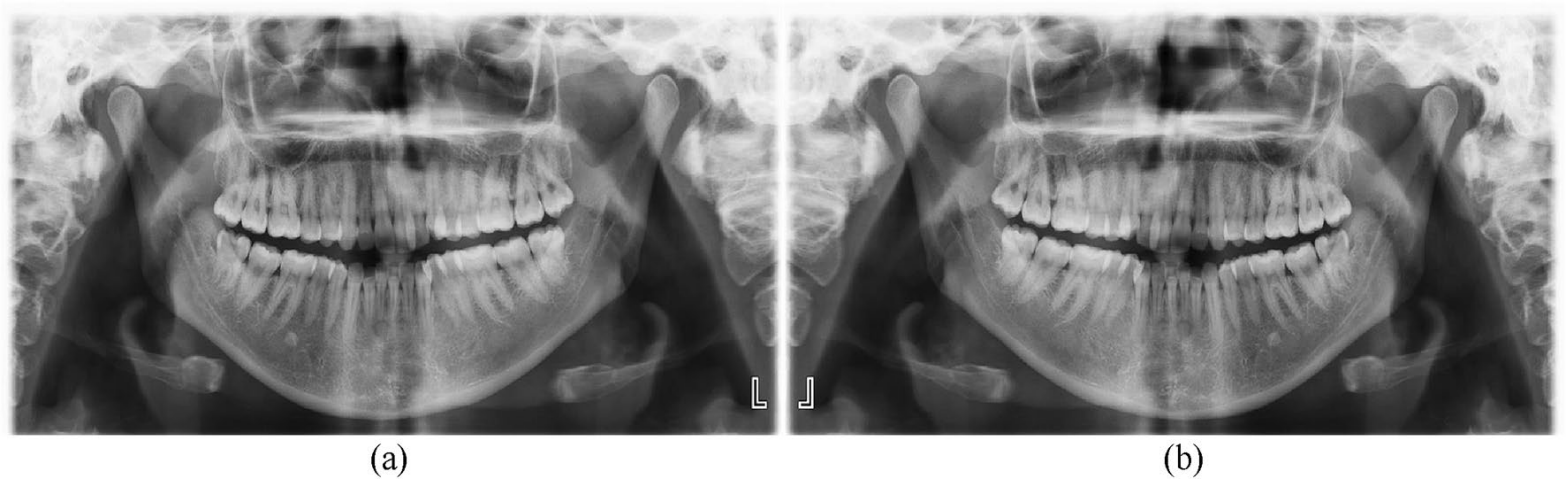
(a) Original panoramic radiograph. (b) Horizontally flipped panoramic radiograph.
